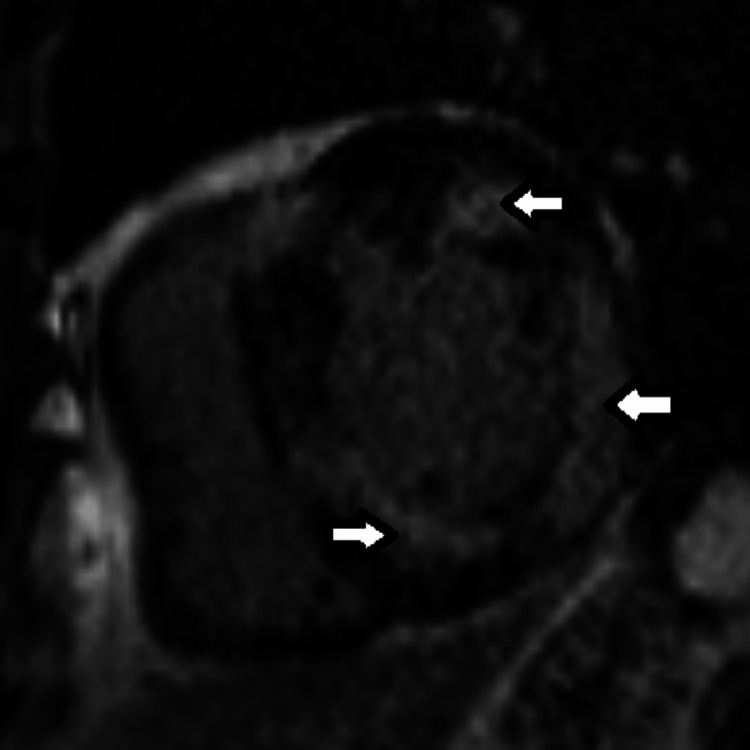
Cardiac MRI (Short axis). Multiple areas of pathologic late gadolinium enhancement with mid myocardial and epicardial pattern with near complete transmural involvement (arrows).
